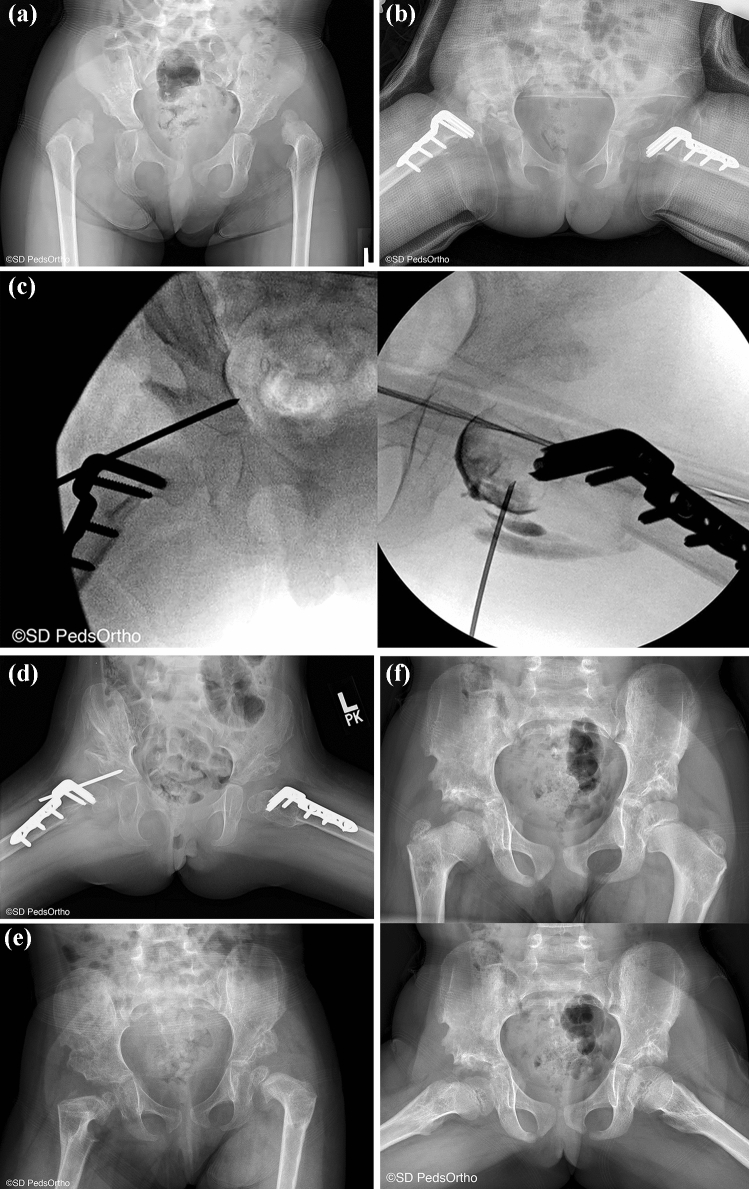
A A 3-year-old female with bilateral hip dislocations. B She was treated with bilateral open reduction and femoral shortening procedures. C The right hip was found to be dislocated posteriorly and was taken back to the operating room and a repeat open reduction was performed, along with a capsulorrhaphy and the hip we held in place with a k-wire. D Six weeks post k-wire fixation. E Six months post k-wire fixation. F AP and frog lateral X-ray 3 years post k-wire fixation
